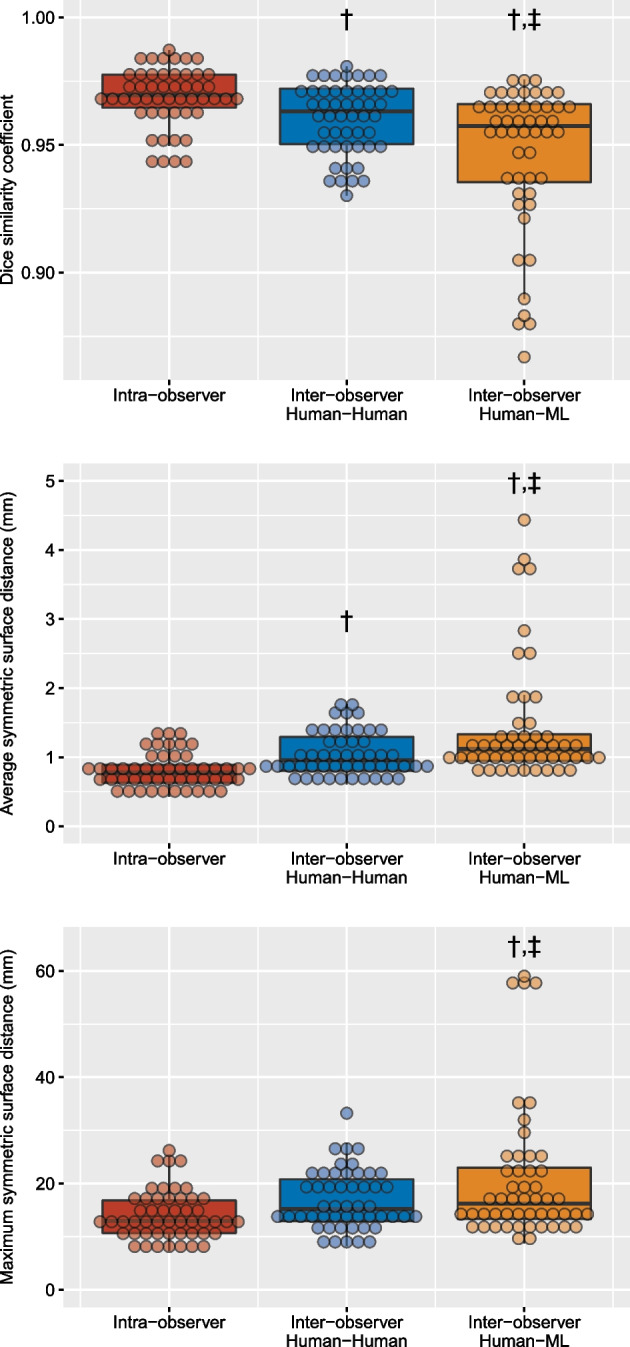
Accuracy of lung segmentation in various conditions (intra-observer, inter-observer human–human and inter-observer human–ML). Each point refers to one computed tomography volume acquisition (four computed tomography volumes were acquired for each patient, i.e., at both end-expiration and end-inspiration at the PEEP level chosen by attending physician, and at end-expiration at PEEP 5 and 15 cmH_2_O). ^†^*p* < 0.05 vs intra-observer, ^‡^*p* < 0.05 vs inter-observer (human–human). ML denotes machine learning and PEEP positive end-expiratory pressure
